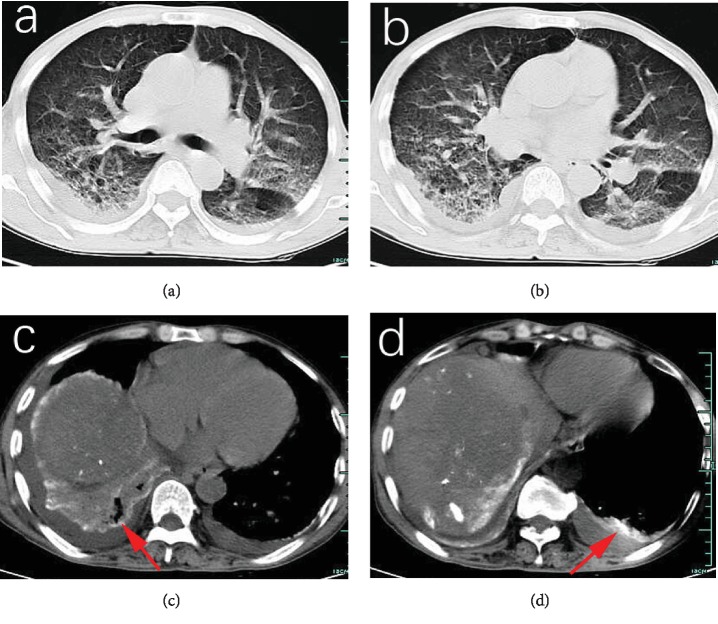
Chest CT findings in a 60-year-old man with an underlying disease of COPD. He suffered from dyspnea and hemoptysis 10 h after TACE for HCC. Chest CT showed diffuse pulmonary infiltration in bilateral lung fields (a, b) and accumulation of Lipiodol (red arrows) located in bilateral lung lower lobes (c, d).
